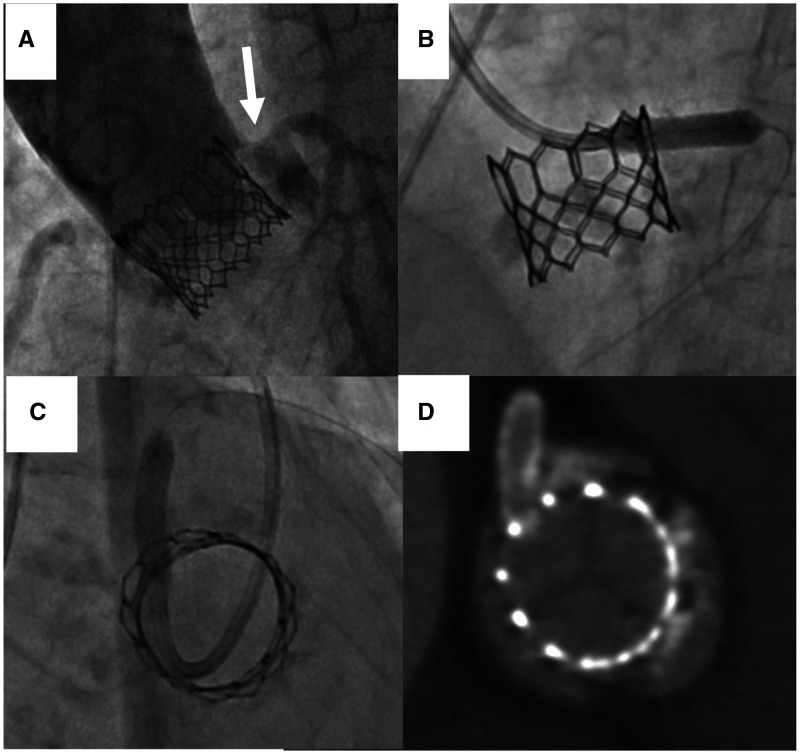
Patient 2: The usefulness of the en face short-axis view for visualizing the positional relationship between the coronary stent and the SAPIEN 3 frame. A left coronary occlusion occurred after SAPIEN 3 implantation. We planned to implant the proximal edge of the stent directly against the SAPIEN 3 frame using a 6 Fr ASAHI Hyperion Judkins Left 3.5 catheter from the left radial artery. The aortogram (*A*) shows calcified native valve tissues occupying the left sinus (arrow). (*B* and *C*) Fluorographic images taken during coronary stent implantation in the perpendicular (*B*) and the en face views (*C*) simultaneously. In contrast to the perpendicular view (*B*), the en face view (*C*) from the deep right-anterior-oblique cranial position (right-anterior-oblique 60°, cranial 42°) shows the positional relationship between the coronary stent and SAPIEN 3 frame. (*D*) Computed tomography imaging after percutaneous coronary intervention shows that the stent was implanted as planned.
